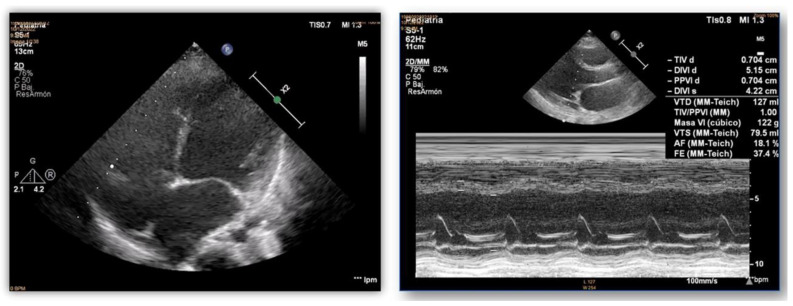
Echocardiograms of index case. Dilatation and poor systolic function of the left ventricle.
